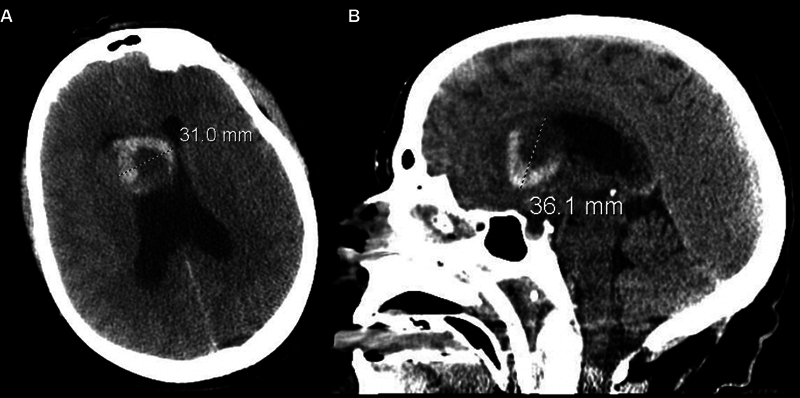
Initial axial (A) and sagittal (B) non-contrast CT head demonstrating acute intraparenchymal hemorrhage of the anterior septum pellucidum and right frontal lobe with intraventricular extension, measuring 31.0 mm by 36.1 mm in size.
